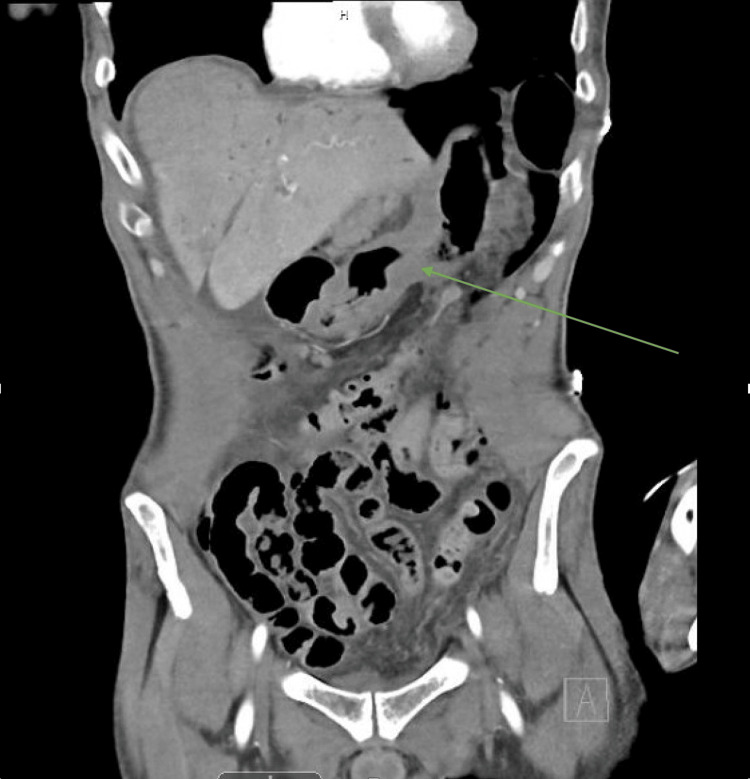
CT scan of the abdomen and pelvis with intravenous contrast Stomach wall thickening is shown by green arrow. CT, computed tomography.
